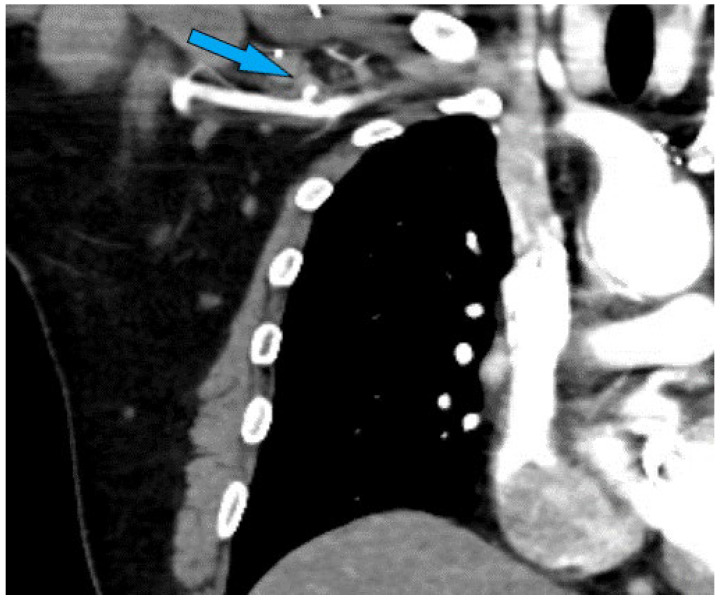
Axillary stump visualized on preoperative CTA indicating previous axillary artery cannulation with end-to-side graft.
